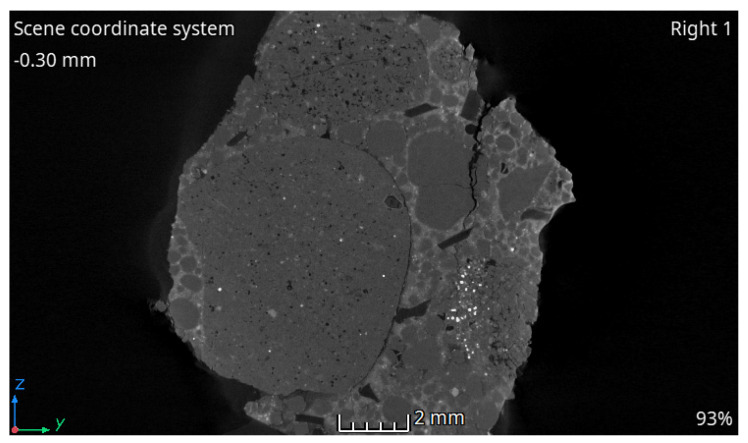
X-ray CT scan—section of a fragment of an SCC-P-15 sample after compression.
